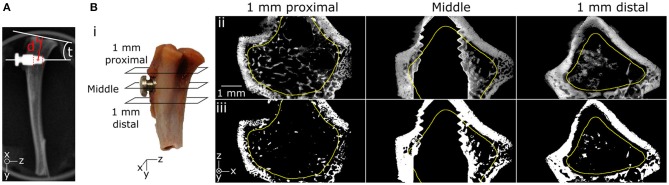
(A) Screw insertion parameters showing distance (d) between the screw and the tibial plateau as well as tilt (t) measured on a 2D radiograph. (B) Trabecular ROI started 1 mm proximal to the screw edge and ended 1 mm distal to the screw edge (i). ROI included trabecular bone and excluded the cortex as drawn in yellow in original (ii) and segmented (iii), as exemplified in three transversal cuts from the unloaded scan.
